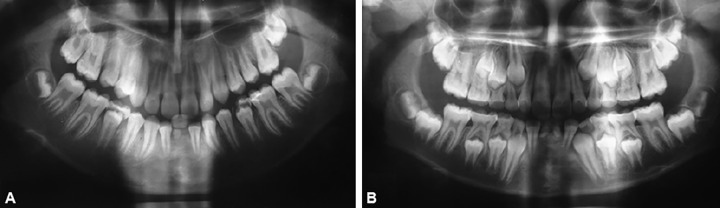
(A) Panoramic radiograph of sibling A, revealing retained left deciduous mandibular central incisor and missing permanent mandibular central incisors and (B) panoramic radiograph of sibling B, revealing retained deciduous mandibular central incisors
